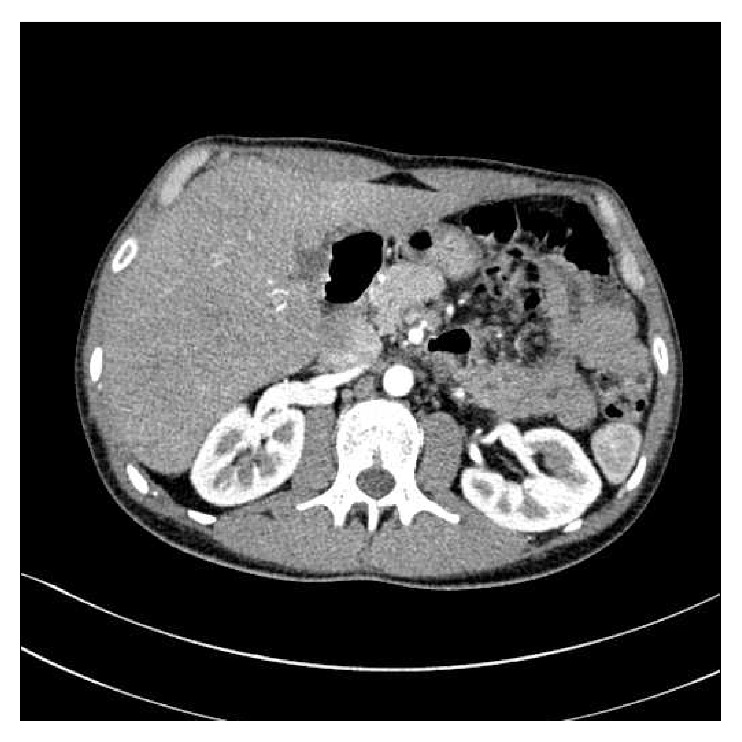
CT scan shows an aspecific thickening of jejunum with partial contrastographic enhancement near the left colon, with a modest dilatation of the lumen of small bowel upstream of the injury.
